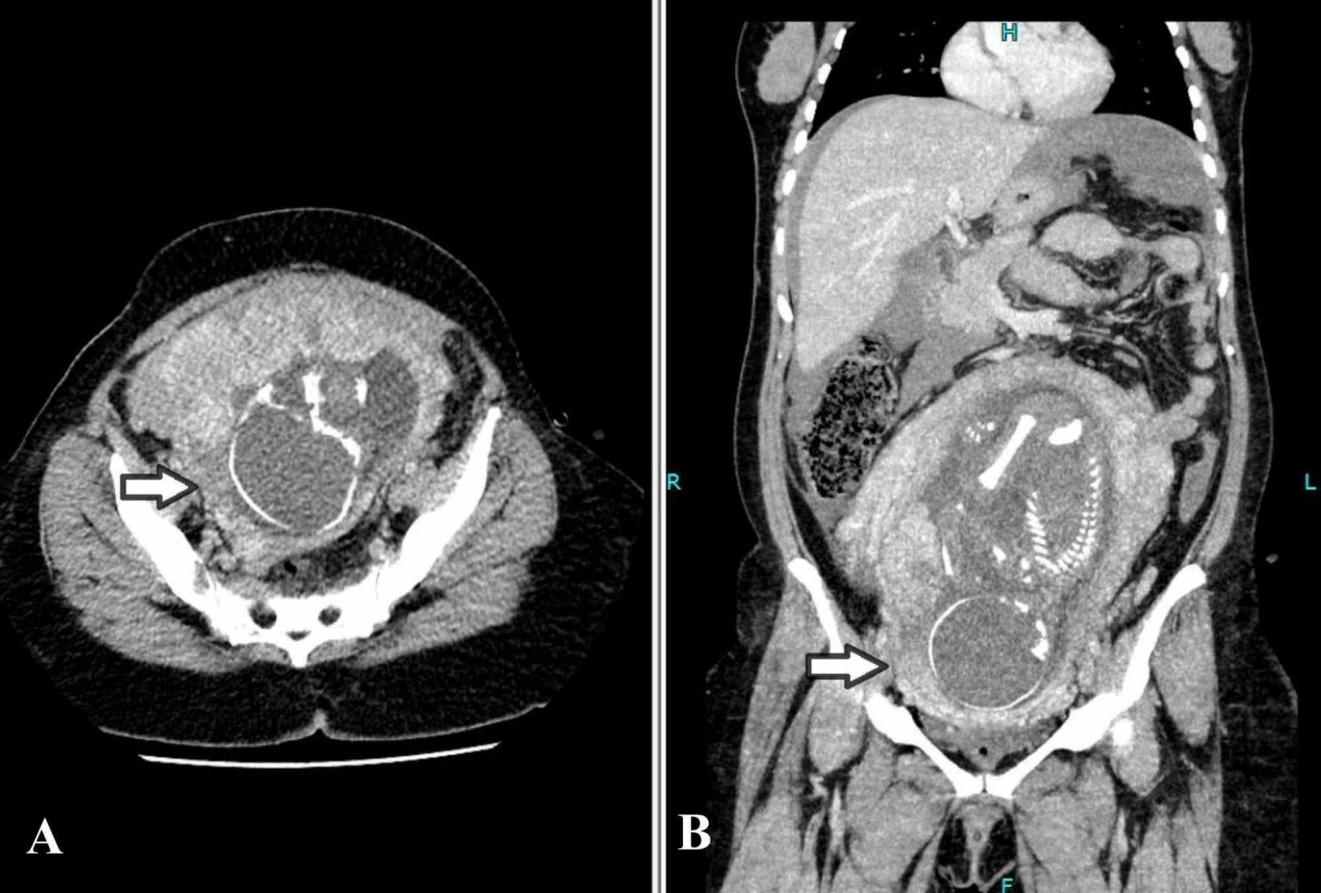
Computed tomography images: (A) axial and B) coronal sections. Gravid uterus with myometrium not clearly appreciated inferolaterally on the right side at site of placental attachment, which was raising the possibility of placenta accreta with uterine rupture (arrows).
